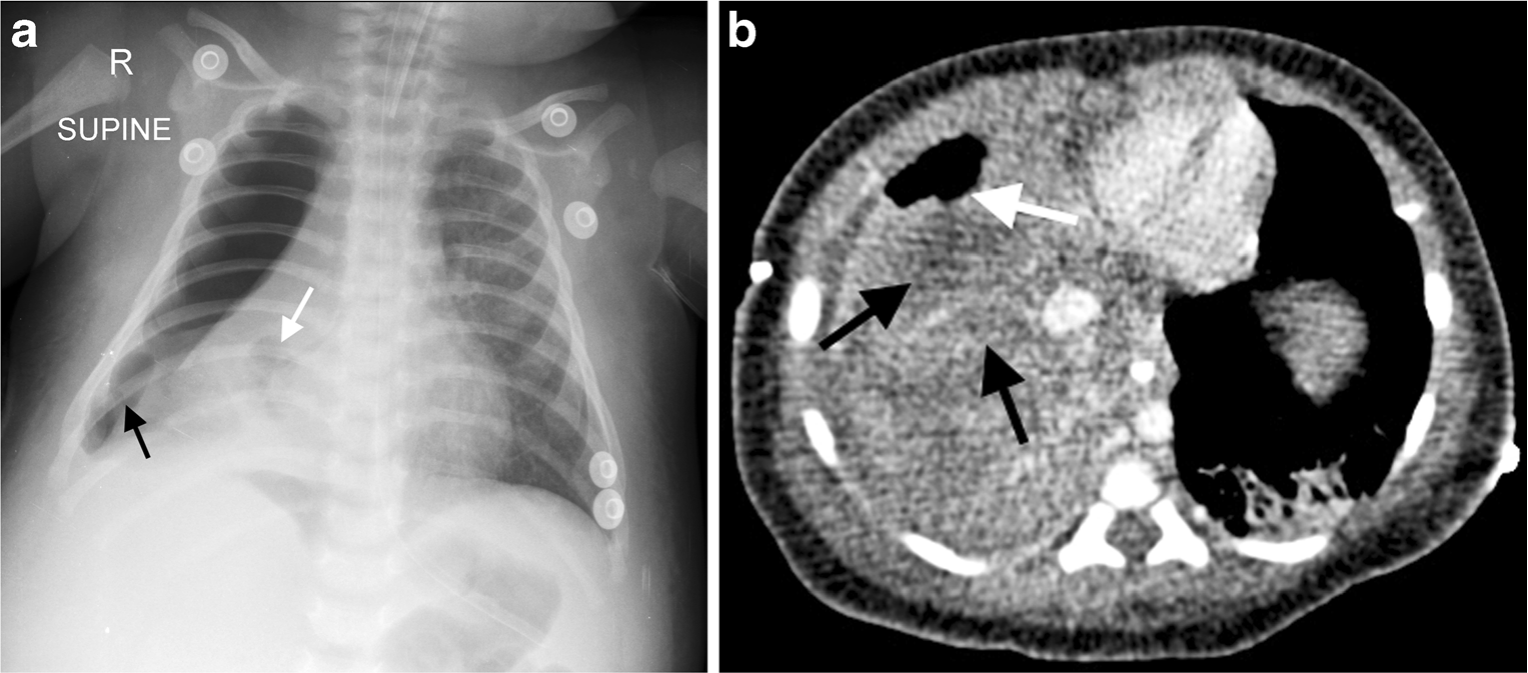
A 3-month-old boy not responding to antibiotics for presumed pneumonia became acutely unwell, short of breath and required intubation a The chest radiograph demonstrates dense collapse of the right lung with suspected cavitation (*white arrow*), as well as loculated gas in the pleural space (*black arrow*), suggesting a bronchopleural fistula. b Contrast-enhanced axial CT of the chest demonstrates non-enhancing areas of the right lung representing necrotising pneumonia (*black arrows*), and a peripheral cavitated portion of the right middle lobe communicating with the pneumothorax (*white arrow*), in keeping with a bronchopleural fistula
